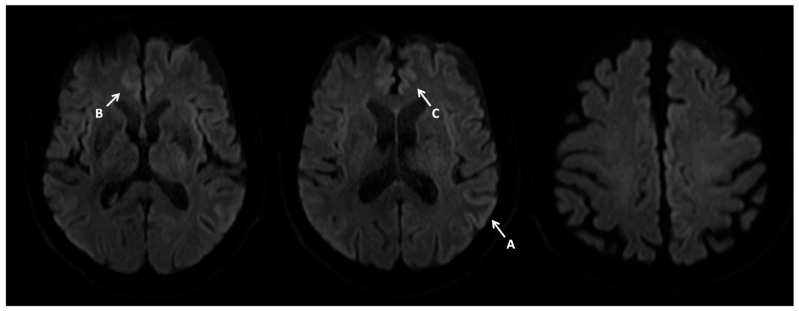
Contrast MRI imaging 23 May 2025. Imaging performed on a 3T scanner. Brain MRI shows moderate diffusion restriction in the cortical layer of the lateral part of the left temporal lobe (A), overlapping a small portion of the parietal cortex, with no detectable changes on Flair and T2 images. Slight cortical signal enhancement in the medial frontal gyri on DWI images (B,C), without significant signal reduction on ADC maps.
